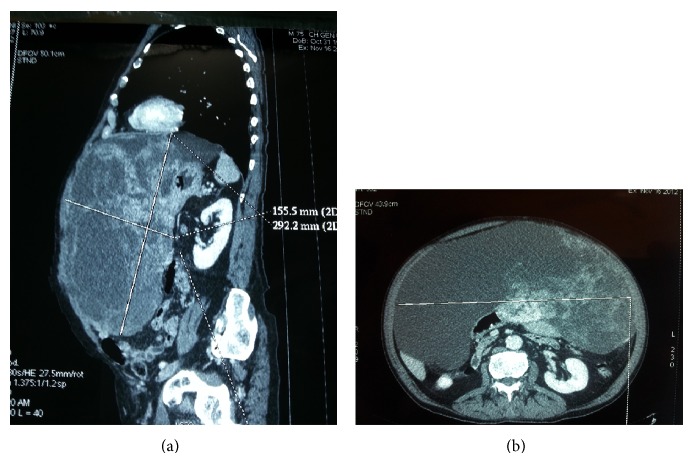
Preoperative contrast-enhanced abdominal CT scan: (a) the sagittal projection shows a large and well-defined, 32 × 29 × 15 cm, heterogeneously enhanced mass that displaces and compresses posteriorly the abdominal organs; (b) the axial projection shows some parts composed of irregular tissue showing enhancement; others are hypodense with cystic aspects and contextual septa.
